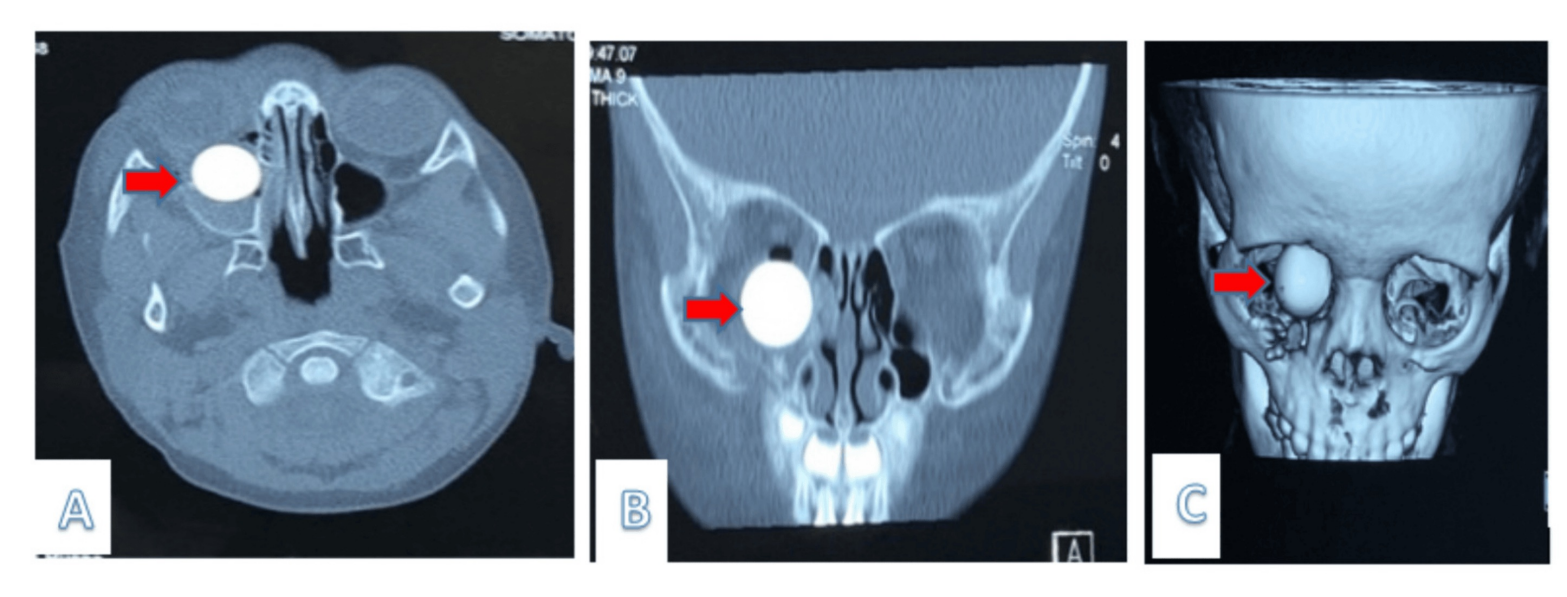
(A) Axial and (B) coronal (C) reconstruction view of a CT scan showing a foreign object spherical in shape, measuring 1.6 (AP) x 1.6 (W) cm, and situated in the right maxillary sinus AP: anteroposterior, W: width
